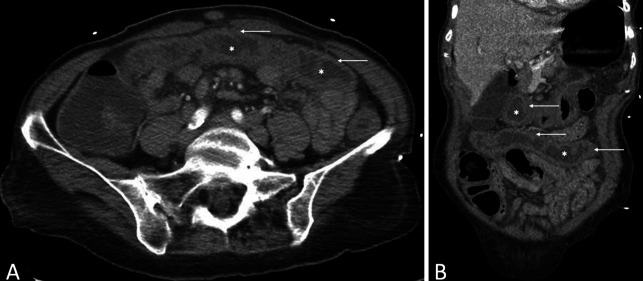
A (axial section) and B (coronal section): gray attenuation pattern indicating pancolitis. Contrast-enhanced computed tomography scans show the distended fluid-filled bowel (asterisks in A and B) and diffuse thickened hypoattenuating bowel wall (arrows in A and B), indicating an inflammatory process without perforation.
